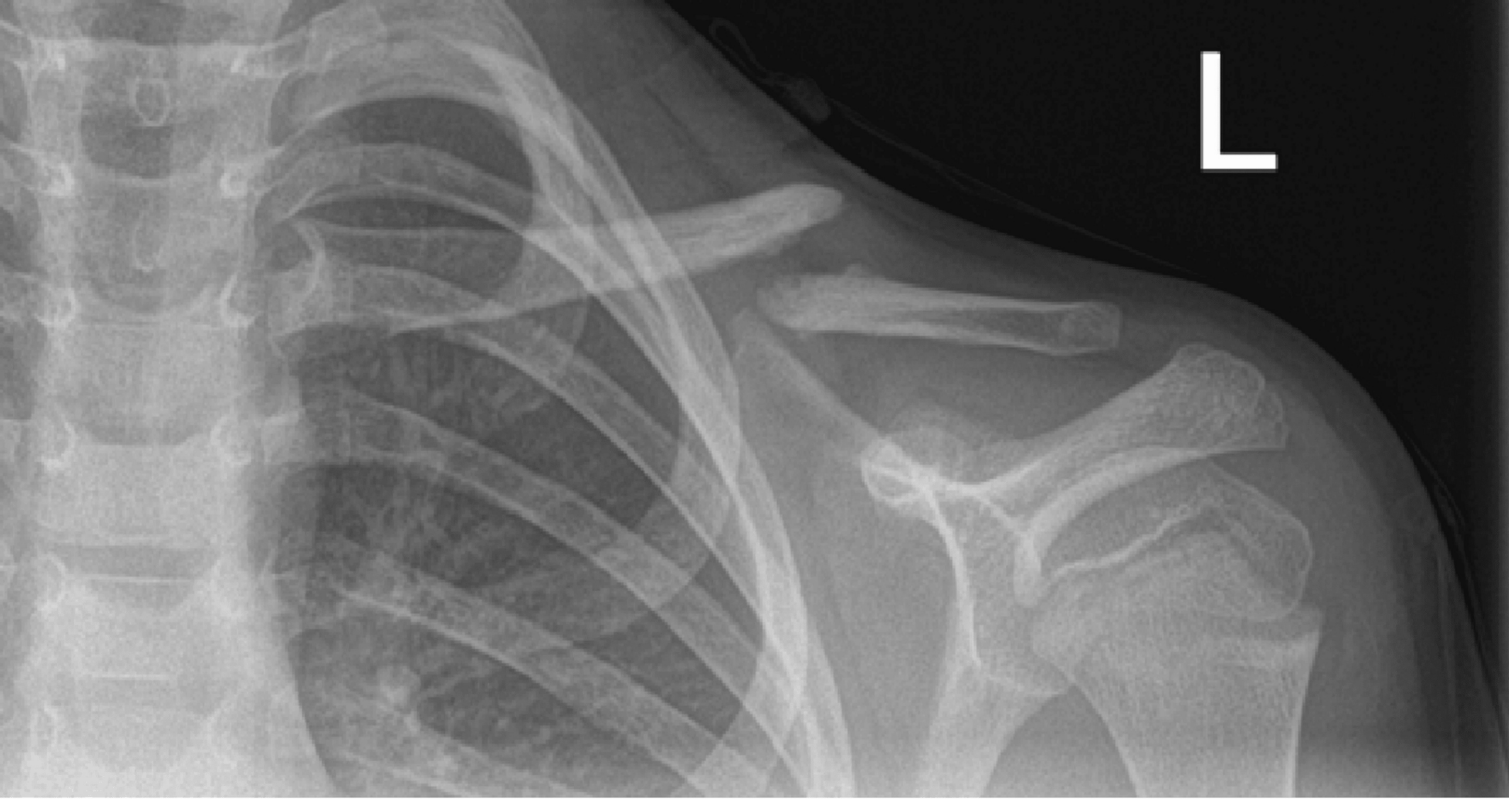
Anteroposterior radiograph demonstrating an atrophic non-union of the left clavicle (Case 2)
